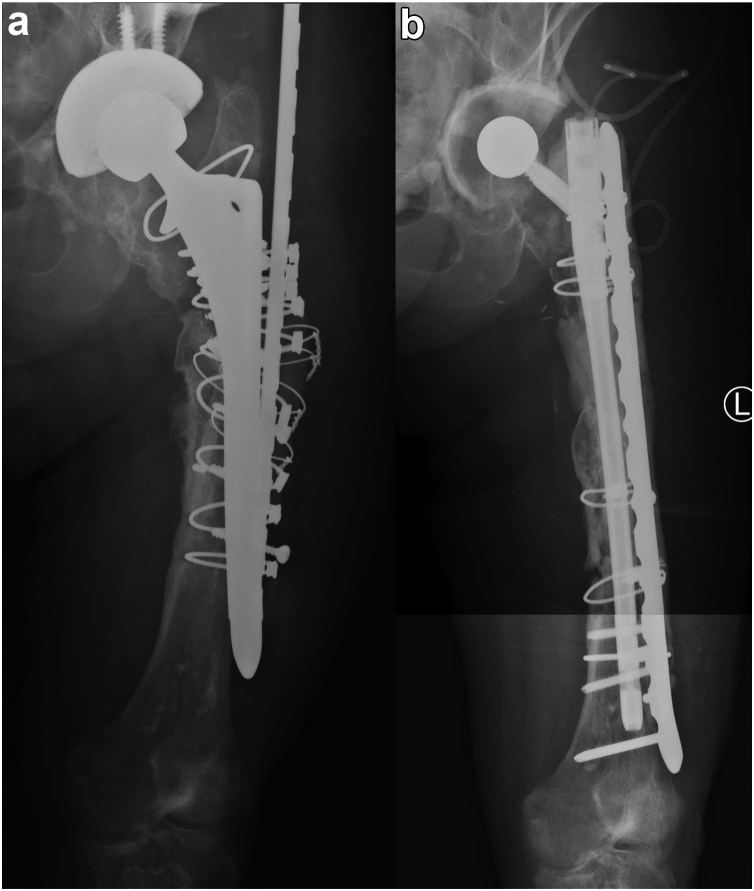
Case 2 QMH (a) Anteroposterior (AP)s X-rays before the first stage revision. (b) AP radiographs after the first stage reconstruction of the femur using a gamma nail and lateral femoral plate coated with antibiotics-loaded cement.
